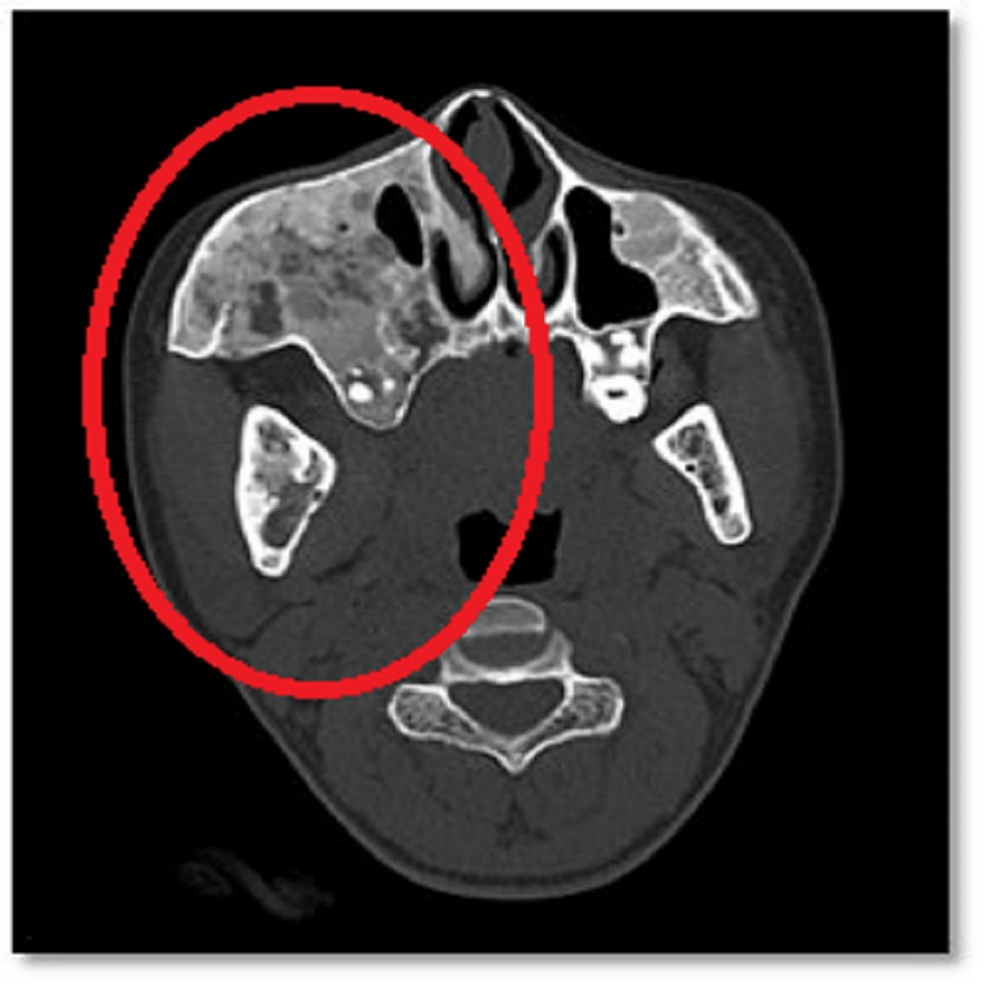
CECT head (axial cut) showing diffuse ill-defined expansile ground glass lesion involving craniofacial region CECT: contrast-enhanced computed tomography
